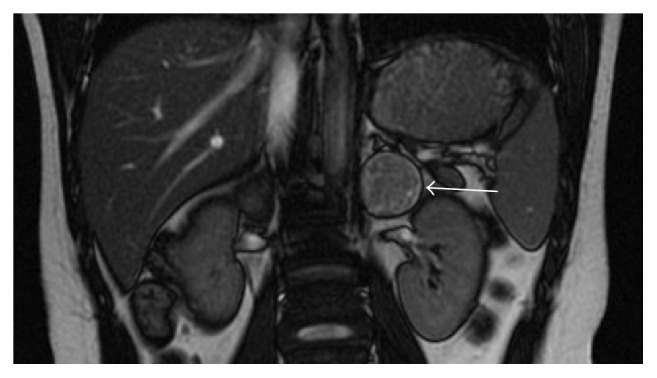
A solid mass (white arrow) 35 × 37 mm in diameter was identified in the left adrenal region in magnetic resonance imaging.
